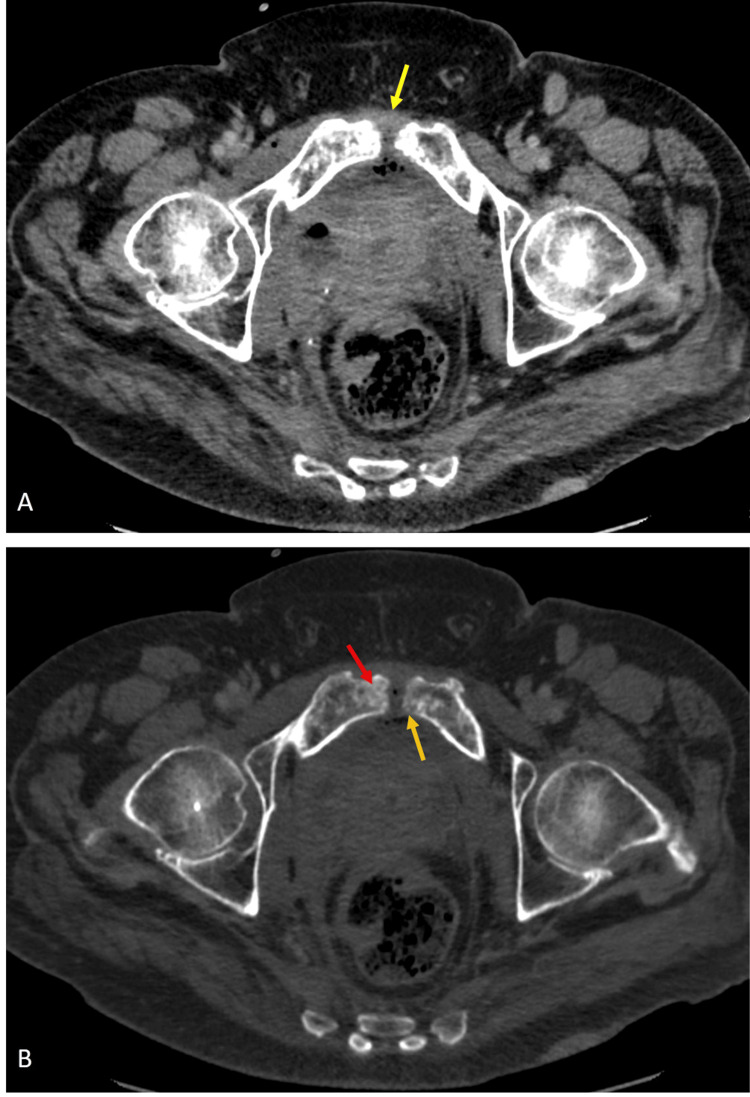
Contrast-enhanced axial CT images through the pelvis in soft tissue (A) and bone (B) windows (A) Contrast-enhanced axial CT images of the pelvis show a small volume of fluid and air locules at the symphysis pubis (yellow arrow), (B) in addition to bony erosions/destruction of the pubic body consistent with osteomyelitis (red arrow).
